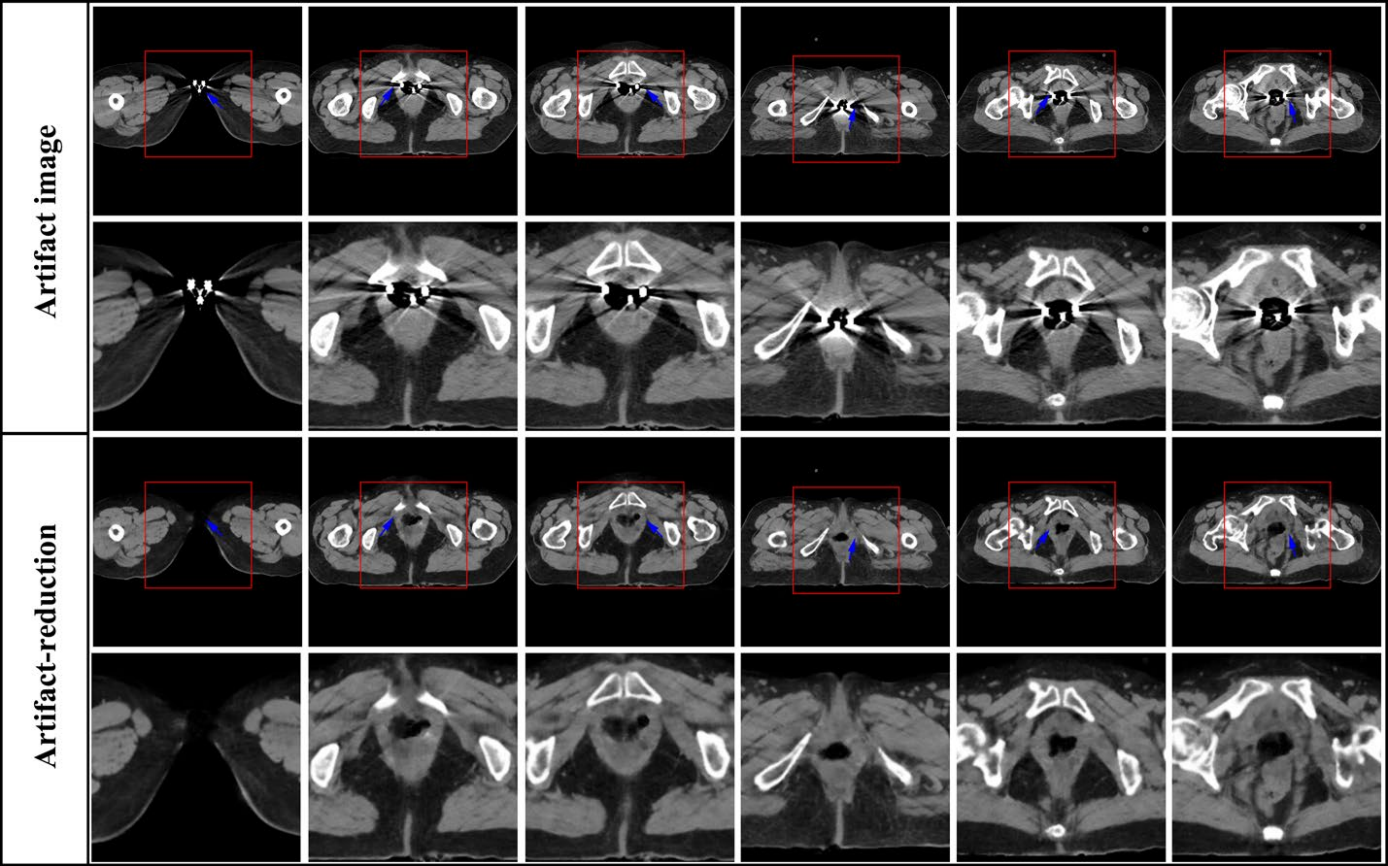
The result of metal artifacts reduction for the clinical artifact cervical CT image caused by intracavitary brachytherapy. The first row is CT images with large metal artifacts, the third row is metal artifacts reduction images by using the proposed method. The second and fourth row correspond to the enlarged area of the red boxes in the first and third row
